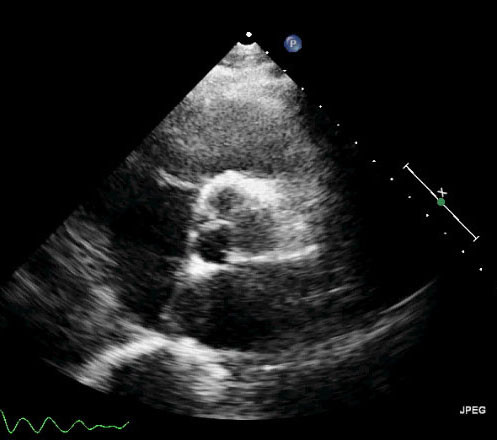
Transthoracic echocardiographic scan (parasternal short-axis view of aortic root) 4 years ago. No tumor was revealed in the right ventricle.
